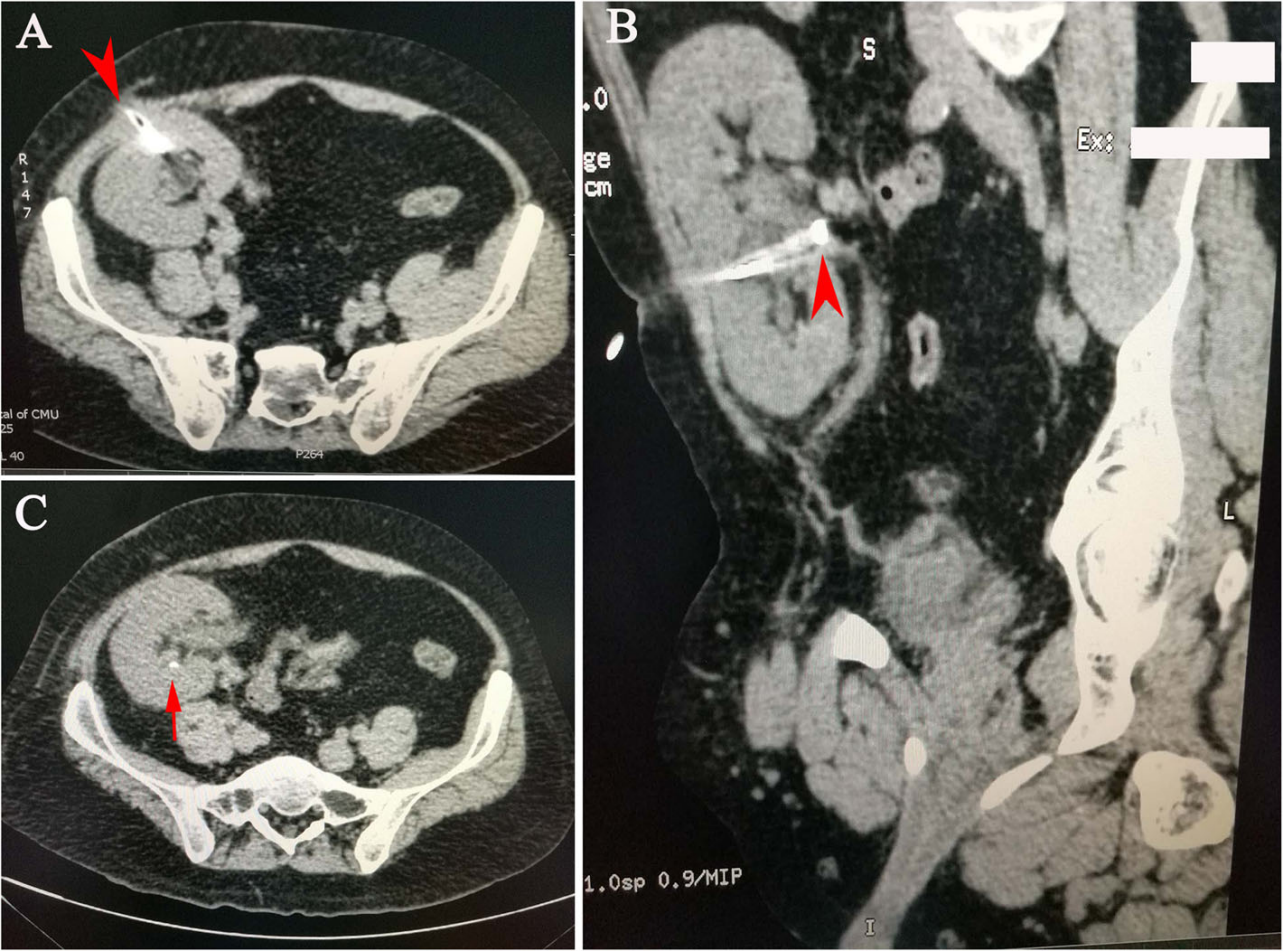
Postoperative CT scan showing a residual calculus in the lateral calyx. a Transverse plane. The red arrowhead shows the percutaneous nephrostomy tube, b Longitudinal plane. The red arrowhead shows the percutaneous nephrostomy tube, c Transverse plane. The red arrow shows the residual calculus
